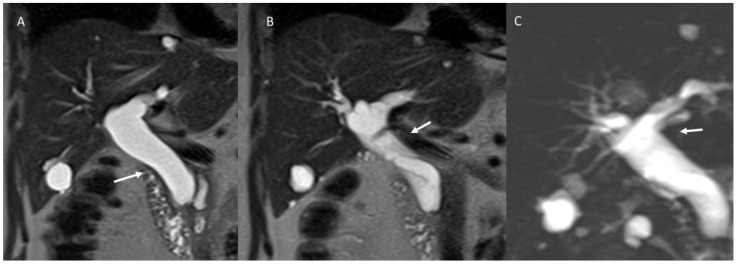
MRI of a 64-year-old male patient with Choledochal cysts. (A,B): T2-weigthed sequences in coronal plane; (C): 3D Magnetic resonance cholangiopancreatography. White arrows show dilatations of the extrahepatic biliary tree.
